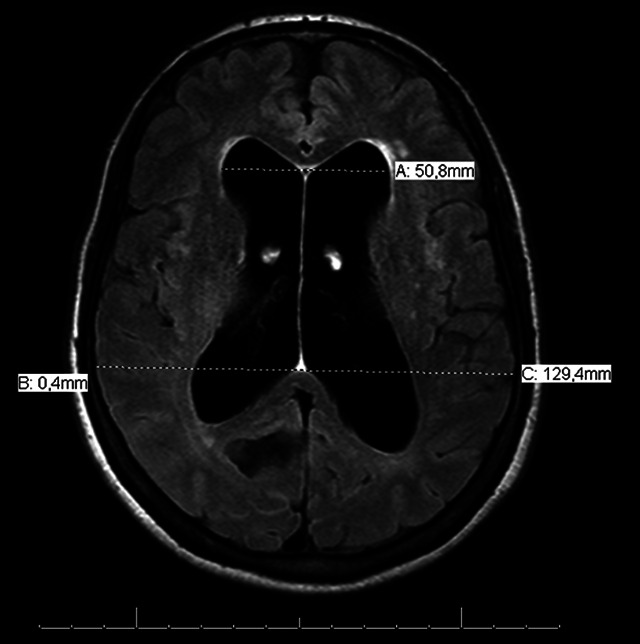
Axial view on FLAIR (*Fluid-Attenuated Inversion Recovery*) sequence showing important ventriculomegaly and high Evans Index, calculated by the reason of bilateral ventricular frontal horns greatest diameter over the greatest skull diameter (estimated as > 0,3 on this example).
